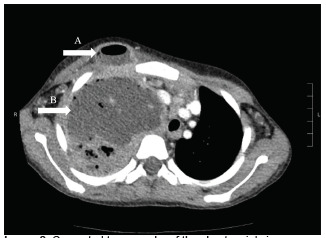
Computed tomography of the chest, axial view, demonstrating massive right hemithorax of multiloculated fluid (B) with extrathoracic fluid and air collection (A) at right anterior chest wall.
